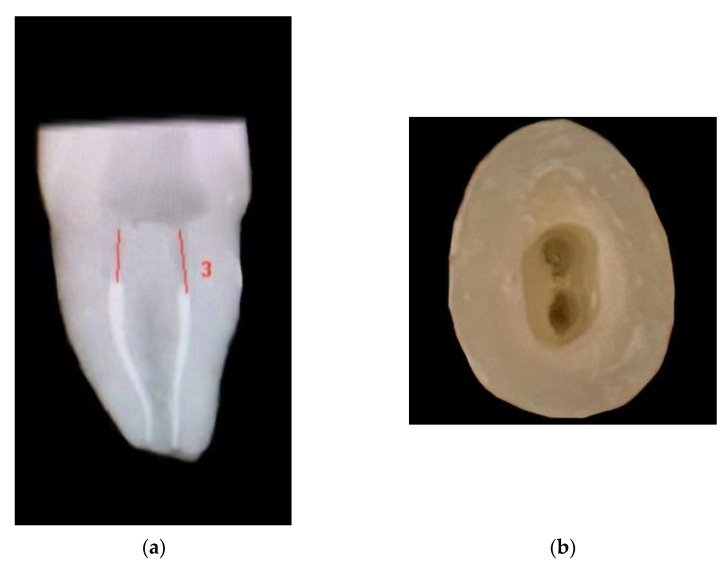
(a) Radiographic proximal view of Endocrown preparation with 3 mm intraradicular extension. (b) Occlusal view of Endocrown preparation wit 3 mm intraradicular extension.
